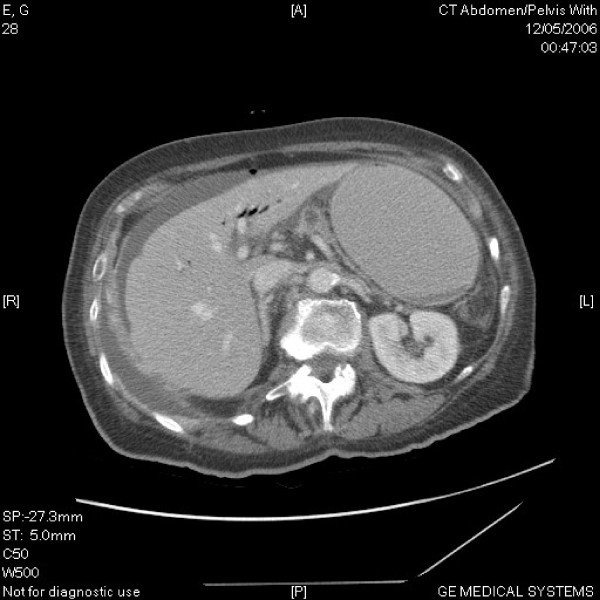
CT abdomen showing air in the biliary tree and free air in the peritoneum.
